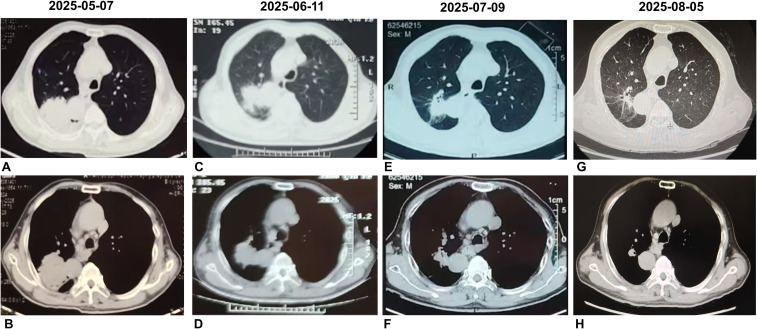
Imaging response to neoadjuvant chemoimmunotherapy. Initial chest CT (A, B) shows a 6.5 × 5.0 cm mass in the right upper lobe with hilar and mediastinal lymphadenopathy. After one (C, D), two (E, F), and three cycles (G, H) of treatment, the tumor progressively decreased in size. Following three cycles, the lesion measured 2.1 cm × 1.7 cm, with marked regression of the mediastinal and hilar nodes.
